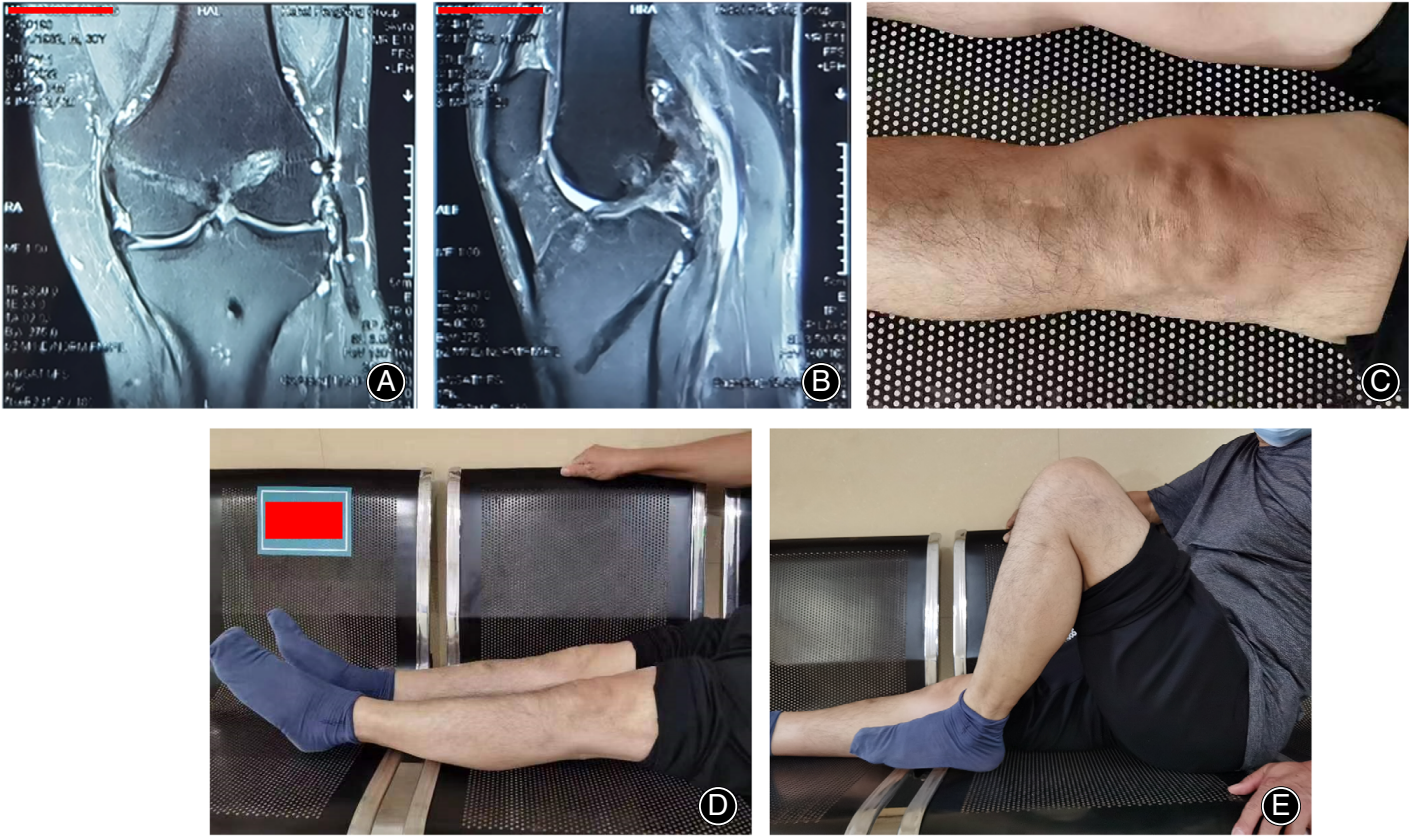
Long‐term follow‐up data. (A, B) Anteroposterior and lateral MRI views of the knee at the 12th year postoperatively. (C) Appearance of incisions 12 years postoperatively. (D, E) The ROM at the 12‐year postoperative follow‐up was satisfactory
